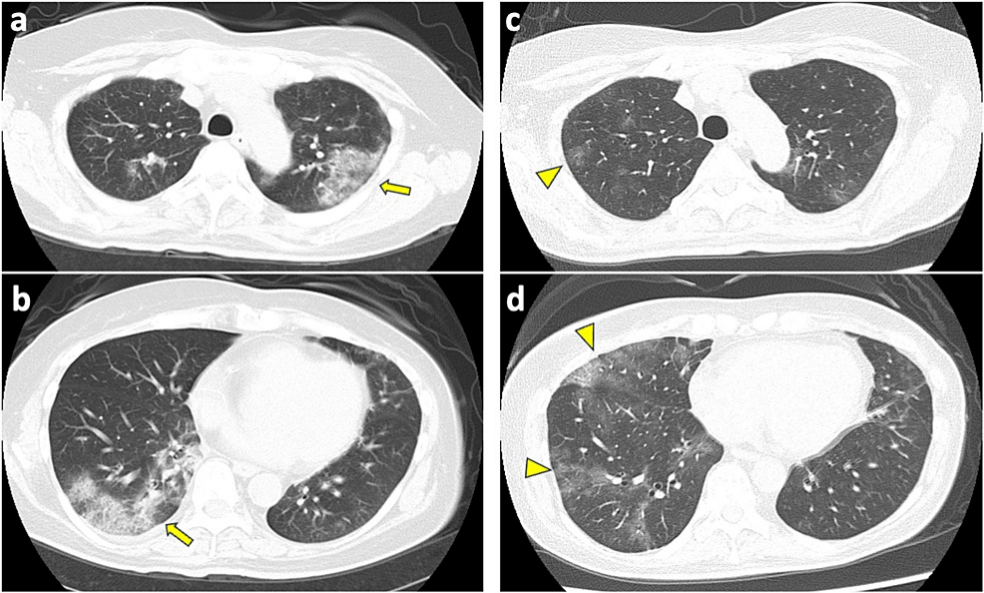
Chest CT images of the patient one month earlier and on admission. Chest CT images of the patient showing (a and b) patchy ground glass with consolidation opacities with reversed halo signs (arrows) in both lungs one month earlier and (c and d) wondering patchy ground glass with consolidation (arrowheads) on admission.
